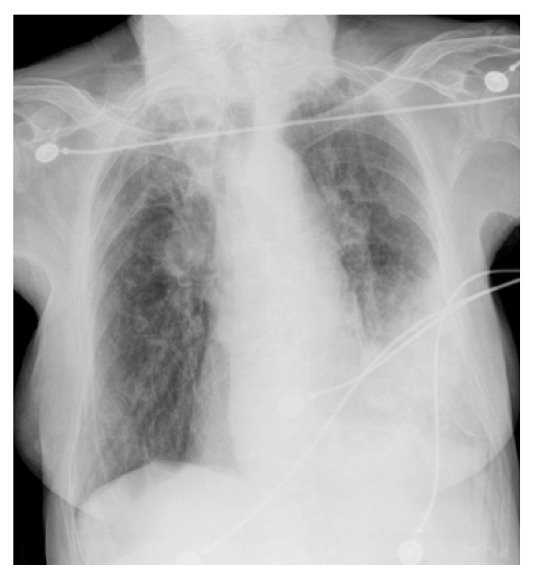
Chest X-ray on admission: left lower lobe alveolar infiltrate.
